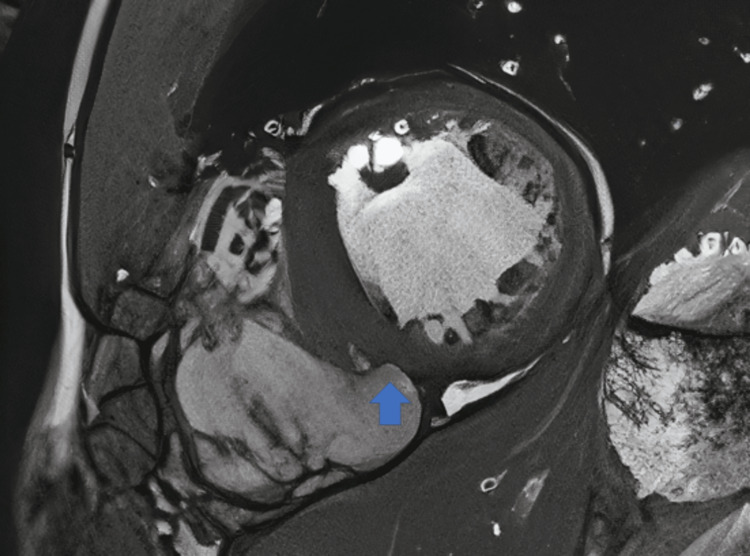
Cardiac MRI featuring focal aneurysm (arrow) measuring 1.9 cm at the infero/septal wall of the right ventricle.
